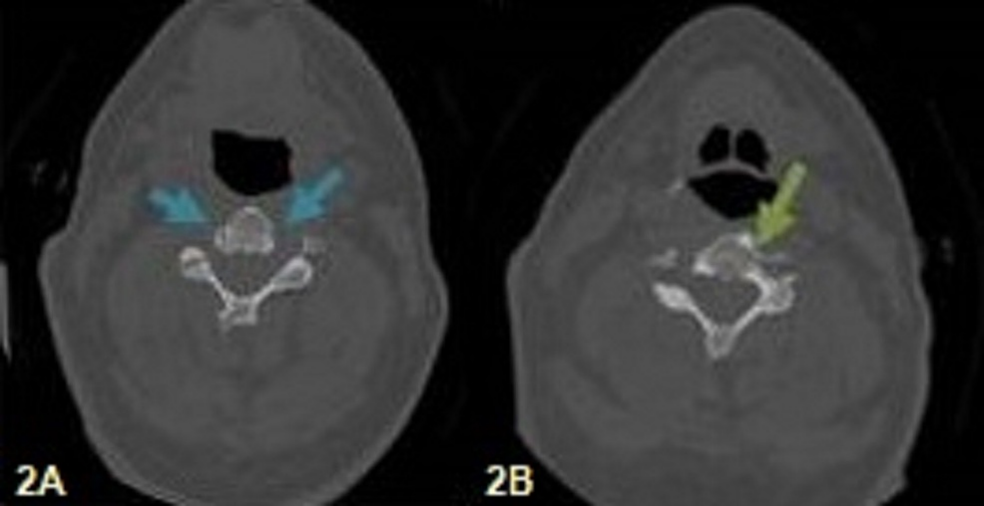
Computed tomography (CT) A 60-year-old man patient with cervical spine trauma who presented with severe pain of the cervical spine, headache, and numbness of his arms: (A) computed tomography (CT) consecutive images of C6 vertebra show the complex of blood vessels like a vertebra's fracture (blue arrows) and (B) CT consecutive images of the C7 vertebra show the blood vessels' abnormality with the course over the left neck of C7 that imitates a fracture (green arrows).
